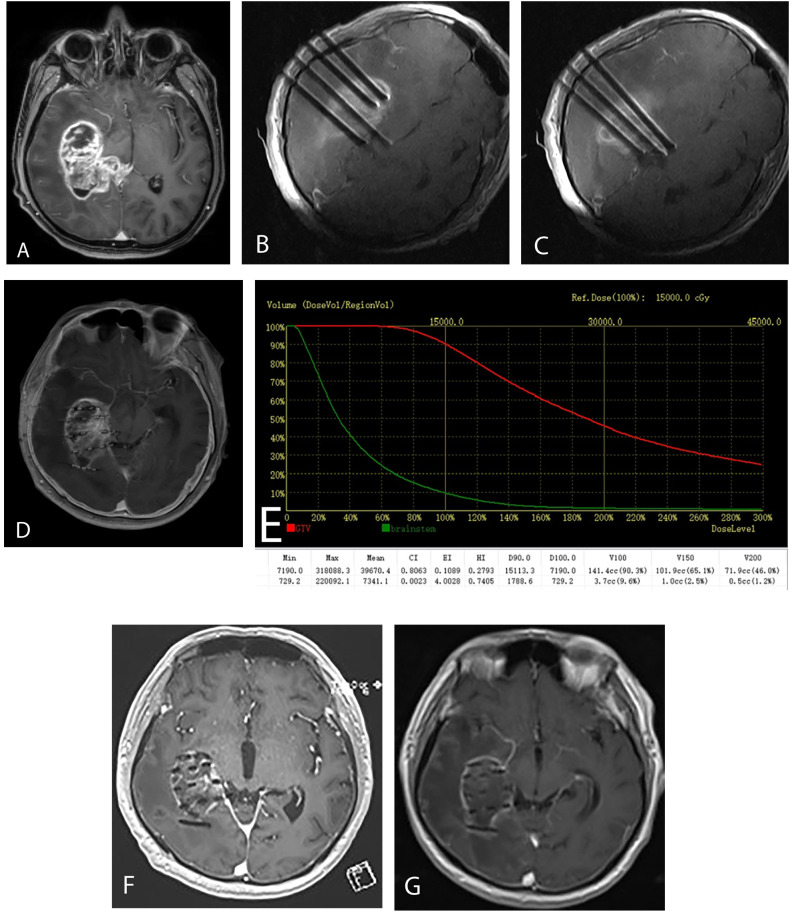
A case of glioblastoma progression after radiotherapy and temozolomide treatment receiving 3D-printed template-assisted ¹²^5^I brachytherapy. (A) Preoperative contrast-enhanced MRI showing tumor invasion into the right lateral ventricle. (B, C) Needles were inserted into the tumor through the template and displayed by MR images. (D) Post-operative CT/MR fusion images. (E) Postoperative dose volume histogram (D90 = 151.1 Gy). (F, G) Contrast-enhanced T1W images were obtained at 6 and 12 months after brachytherapy. These images demonstrate a significant reduction in tumor enhancement along with a marked decrease in mass effect, indicating effective local control.
